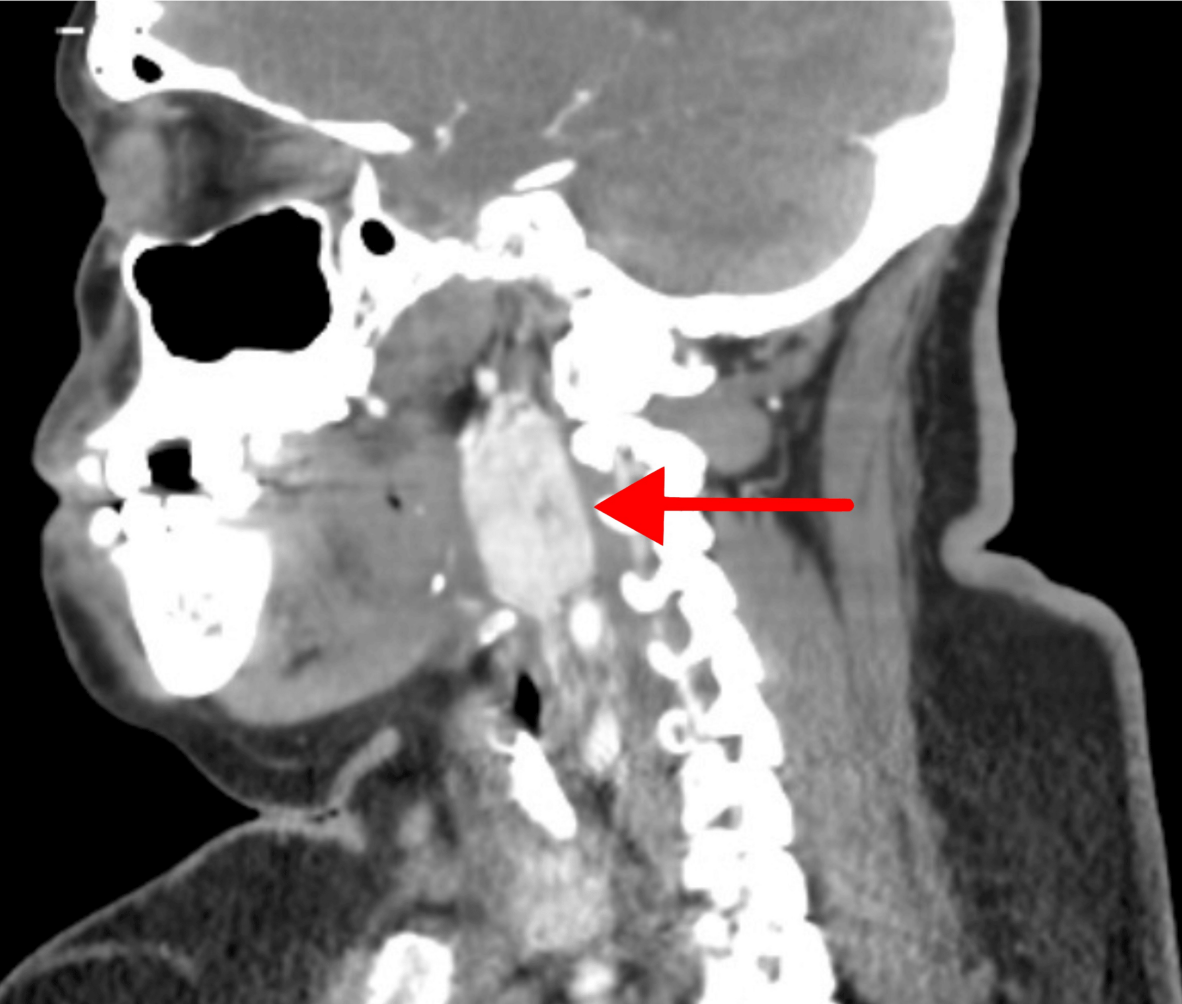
CT angiography showing a sagittal image of a CBT extending to the base of the skull CBT, carotid body tumor
